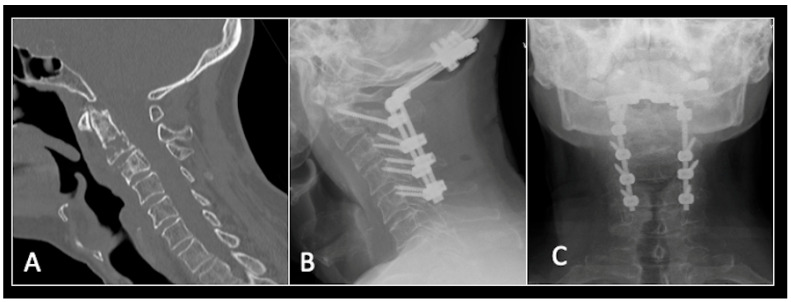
(A) Sagittal CT imaging showing pathological fracture of C2 vertebral body. (B,C) Posterior occipitocervical stabilisation on lateral (B) and AP (C) radiographs, respectively.
